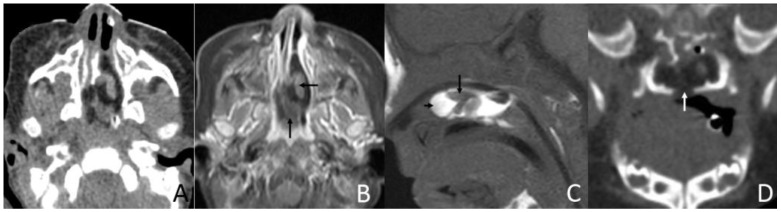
CT and MRI of a 2-month and 17-day girl presenting shortly after birth with dyspnea and inspiratory stridor. (A) Axial CT, displayed in the soft-tissue window, shows the soft palate mass protruding to the left nasal cavity and blocking the bilateral choanae to be of predominantly low attenuation, containing an irregular moderate-attenuation stalk. (B) Axial, postcontrast, T1W-SPIR MRI shows that the lesion’s stalk is slightly enhanced (arrows), but the rest of the lesion is not enhanced. (C) Sagittal T1-weighted MRI shows an irregular mass in the soft palate and left nasal cavity that mainly has a high-signal (short arrow) with an irregular low-signal central stalk (long arrow). (D) Coronal CT, displayed in the bone window, shows the soft palate mass associated with the cleft palate (arrow).
